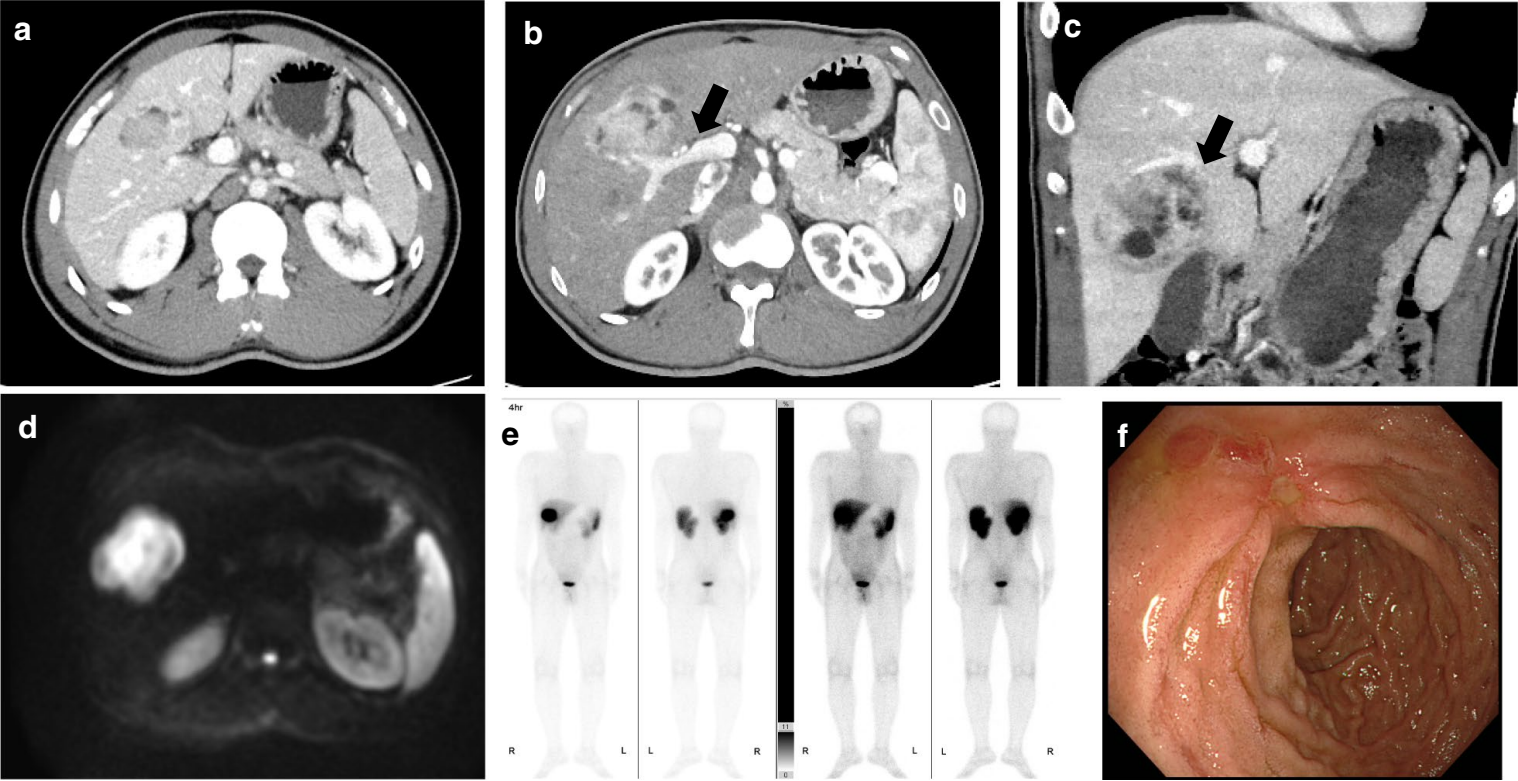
Preoperative imaging. a Abdominal contrast-enhanced computed tomography (CT) showed a tumor at the first visit (55 mm). b Abdominal contrast-enhanced CT showed a hypervascular tumor with cystic degeneration in segment 5/6 of the right hepatic lobe (78 mm). The tumor is in close proximity to the right branch of the portal vein (arrow). c The tumor partly involved the S4a region, and invasion to the middle hepatic vein (MHV) was suspected (arrow). d Magnetic resonance imaging (MRI) showed a hyperintensity on diffusion-weighted imaging. e Somatostatin receptor scintigraphy (SRS) showed abnormal uptakes in the liver. f Upper gastrointestinal endoscopy showed multiple duodenal ulcers (H1 stage)
